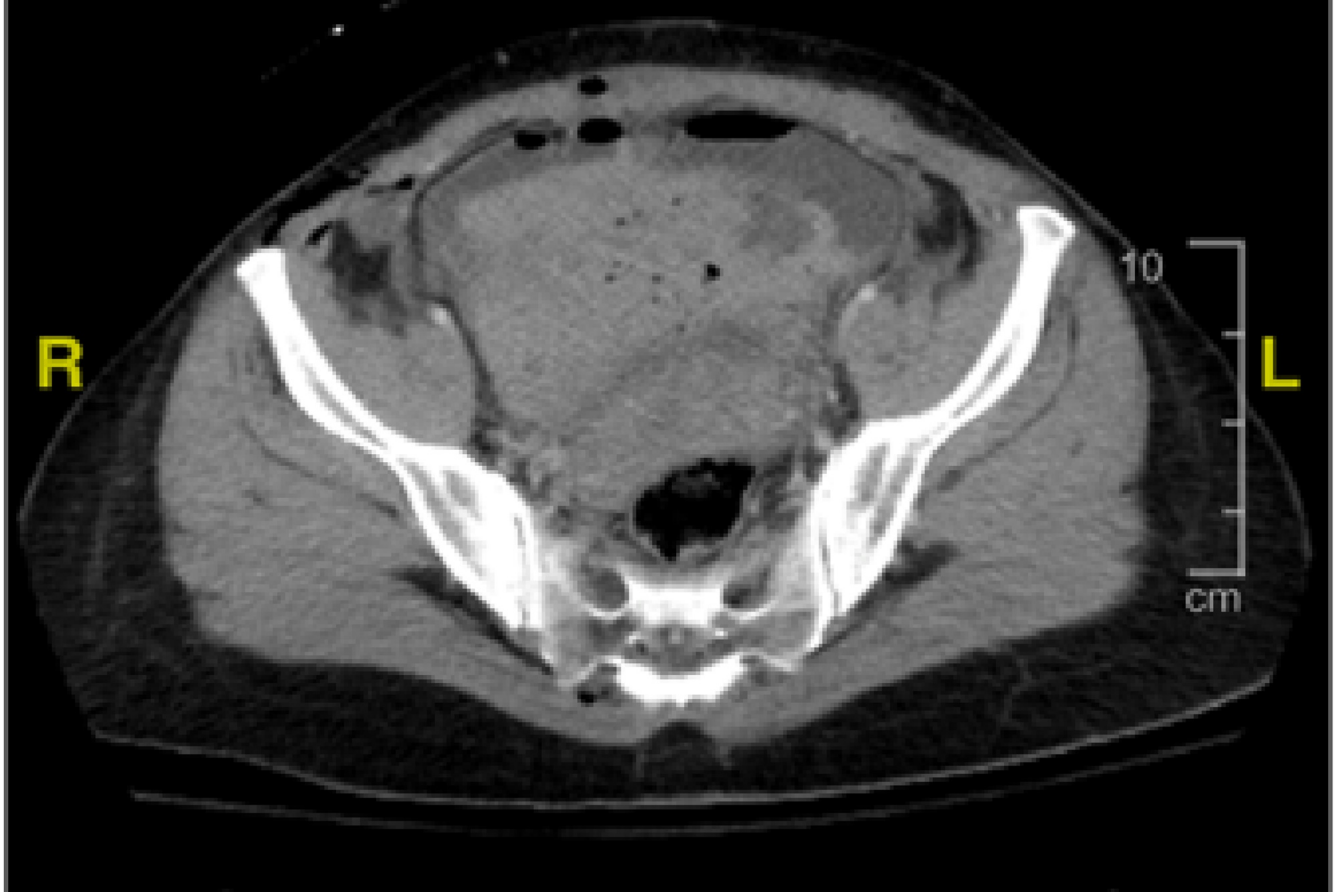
CT scan showing blood clot inside the bladder
